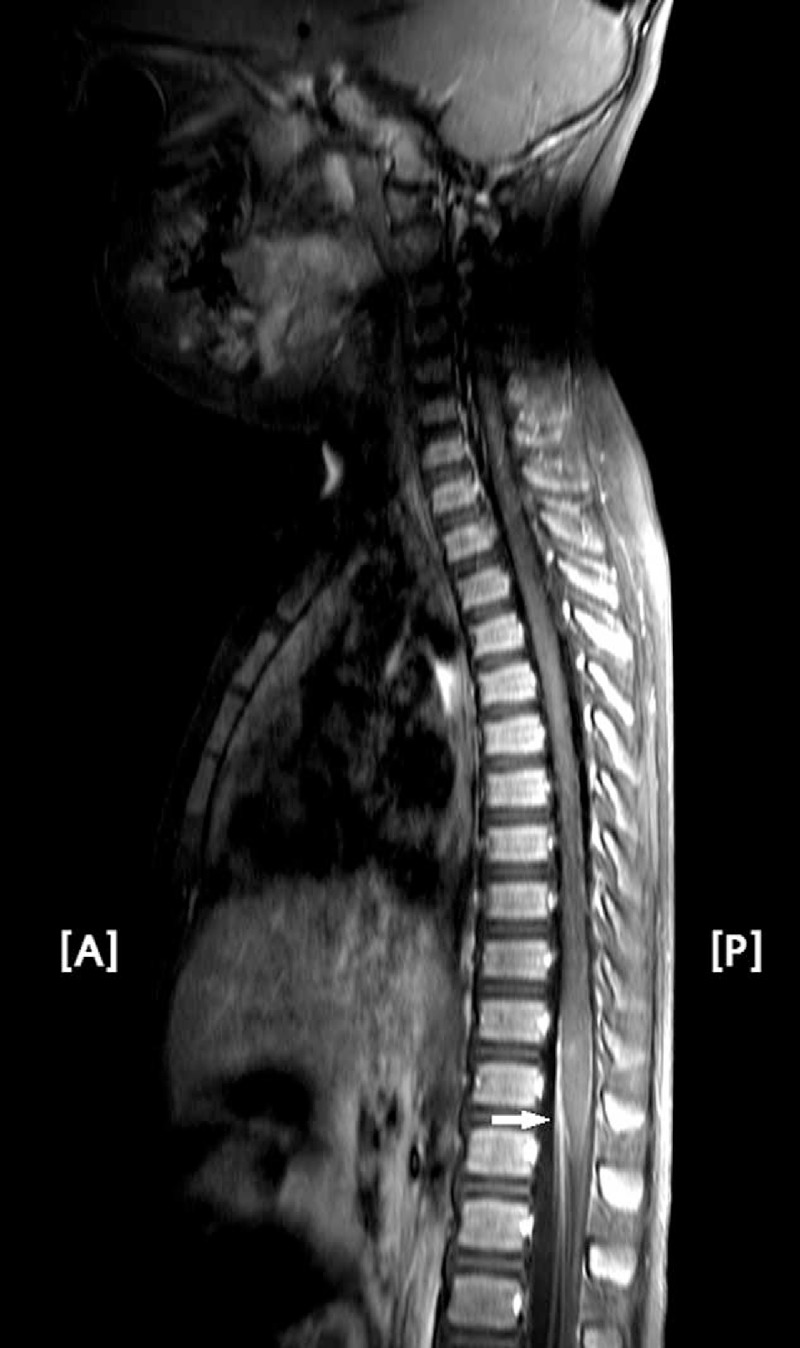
High signal intensity (white arrow) was observed on the cervical and lower thoracic spinal cord as well as the cauda equina in T-1-weighted MRI images after gadolinium injection. A = anterior, MRI = magnetic resonance imaging, P = posterior.
